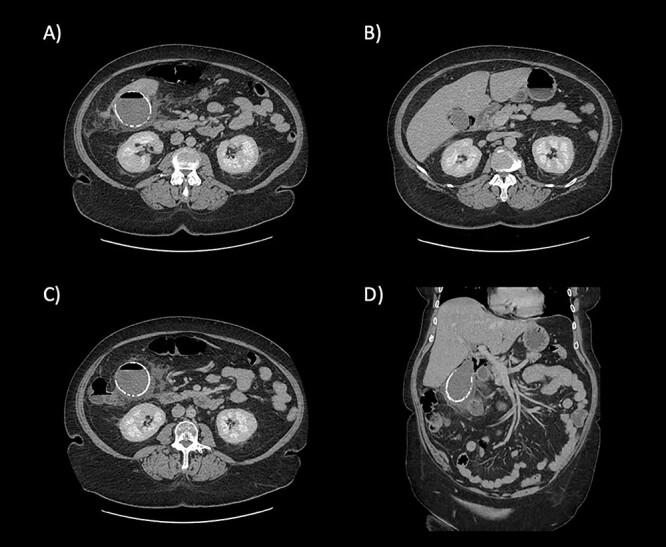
CT scan images demonstrating the calcified wall of the gallbladder consistent with a porcelain gallbladder (A). Pericholecystic fluid and stranding (A and B) demonstrate cholecystitis. The presence of air within the wall and lumen of gallbladder is consistent with emphysematous cholecystitis (C). Air is also seen tracking along the hepatic hilum (D).
